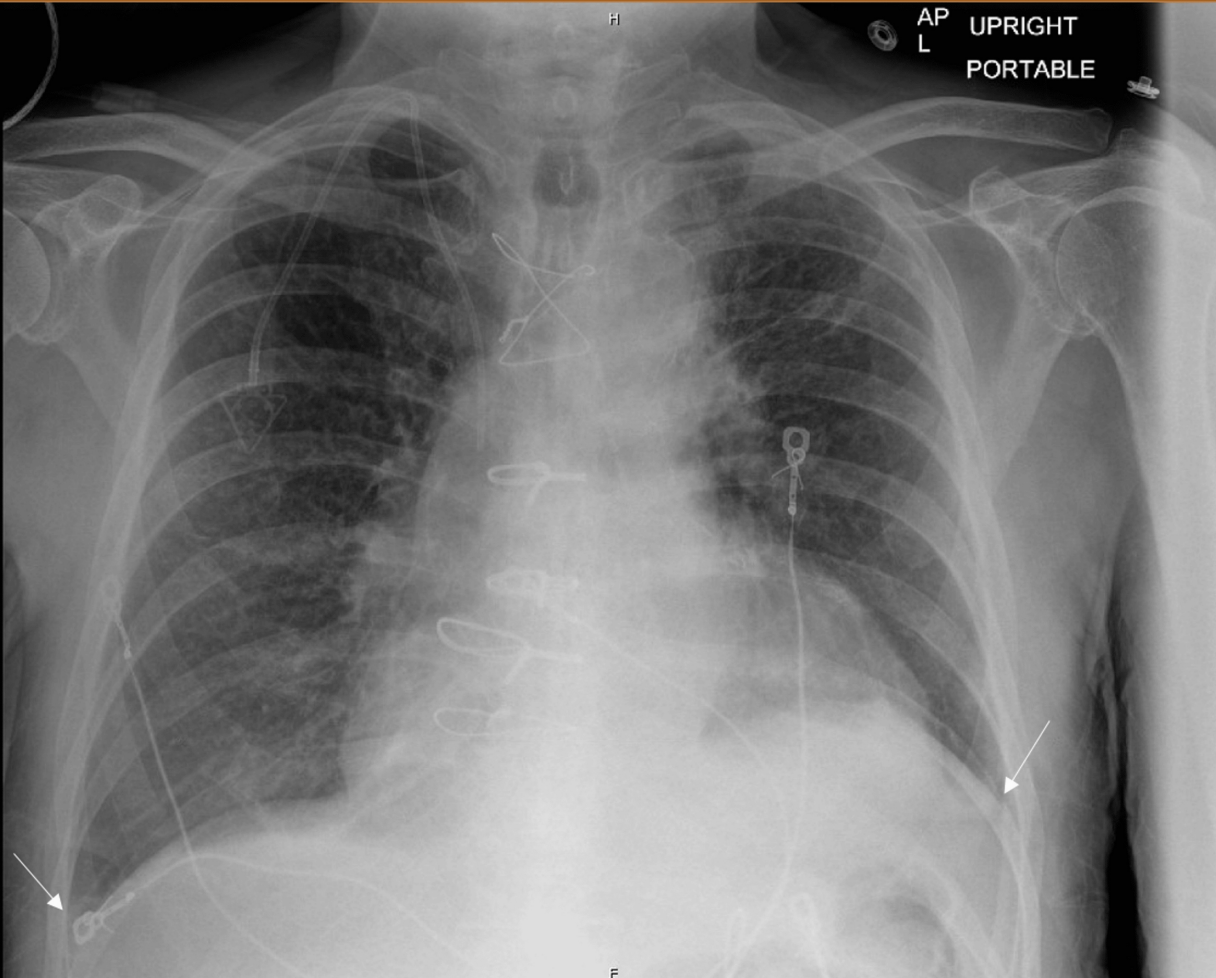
Chest X-ray showing cardiomegaly and pulmonary edema.
